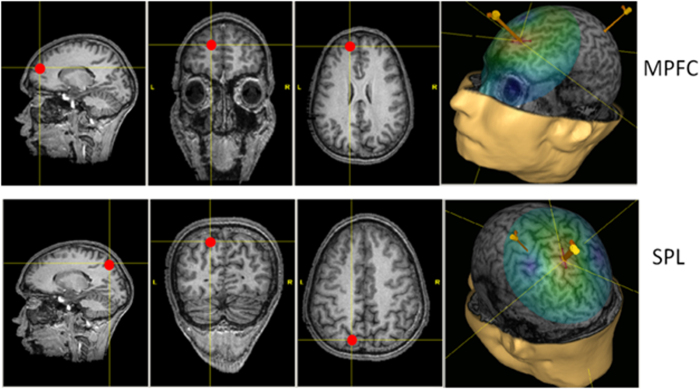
For each stimulation condition, the targeted point is shown on MRI slices by the red dot. An example of the induced electric field represented on a 3D reconstruction of the brain is also shown for each stimulated site, with the hand area in the primary motor cortex as a reference point. For left MPFC, we targeted the area BA10, i.e. anterior part of the superior frontal gyrus, very near the median line. The coil handle pointed backward with an angle of approximately 10° with respect to the midline. For left SPL, we targeted area BA7, i.e. part of the parietal cortex, located between the marginal branch of the cingulate sulcus and the parieto-occipital sulcus. The coil handle pointed towards the back of the head, forming a 45° angle with respect to the midline in the clockwise direction.
